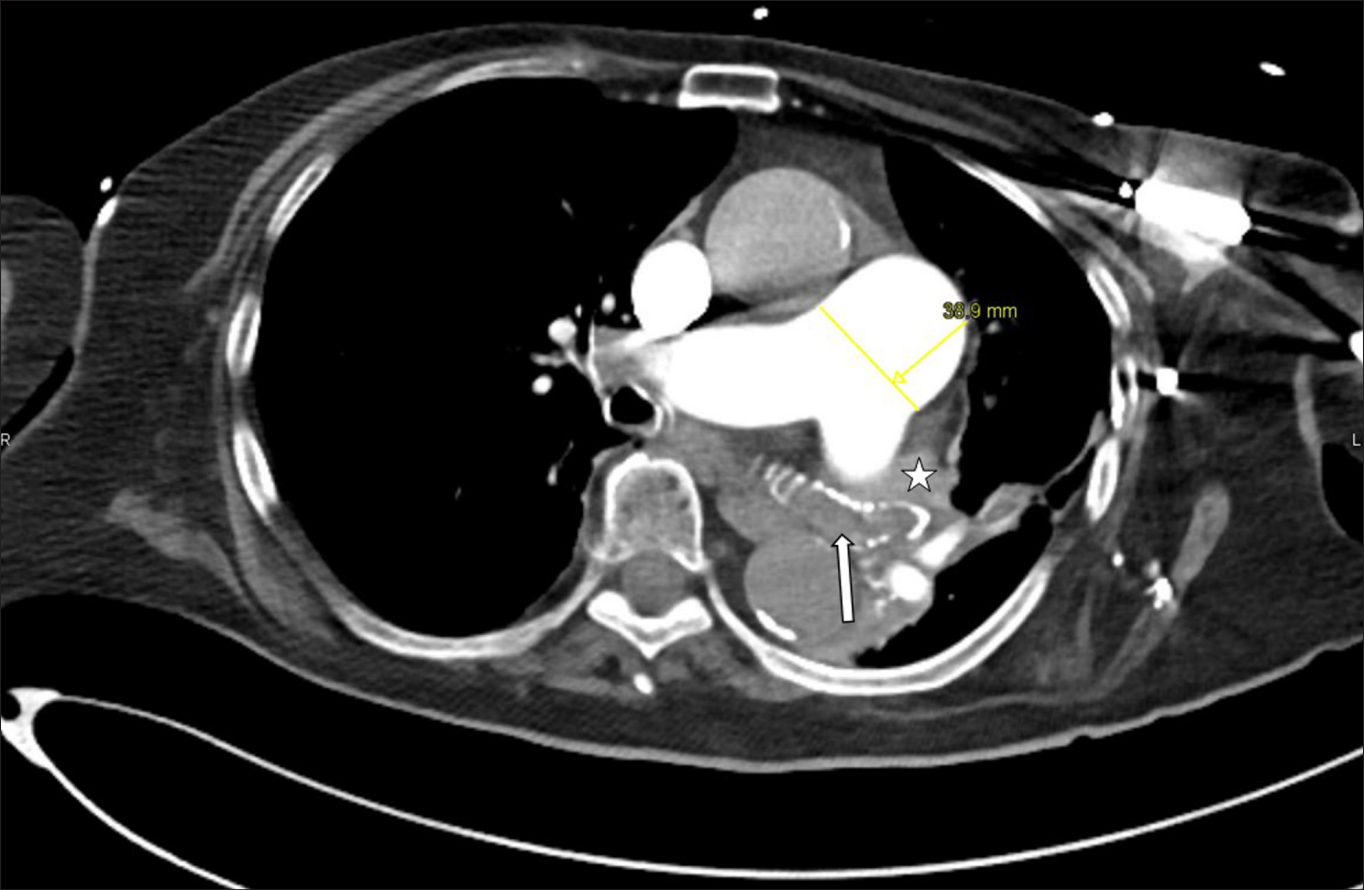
Axial CT of the pulmonary trunk in mediastinal window. Central bronchi on the left side are outlined by calcifications of bronchial walls cartilage and are filled with mucous (white arrow). The left superior pulmonary vein is not opacified (star). The pulmonary trunk diameter is 38.9 mm (normal being ≤27 mm in females), likely because of PA hypertension.
